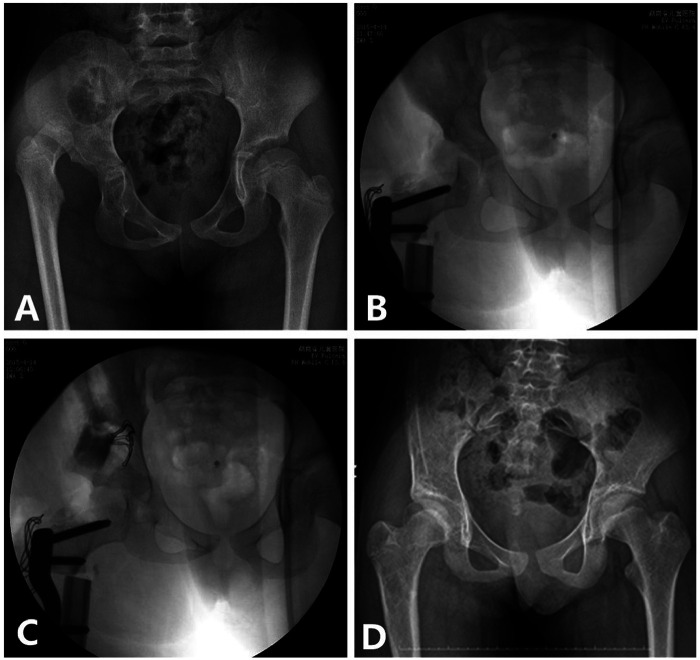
Representative case of MPO for right DDH. (A) Preoperative anteroposterior pelvic radiograph (8y3 m, F) showing hip dislocation and increased AI. (B) Intraoperative fluoroscopy confirming central reduction. (C) Immediate postoperative radiograph demonstrating improved coverage. (D) 6-year follow-up showing maintained reduction, remodeled acetabulum, and no AVN signs.
